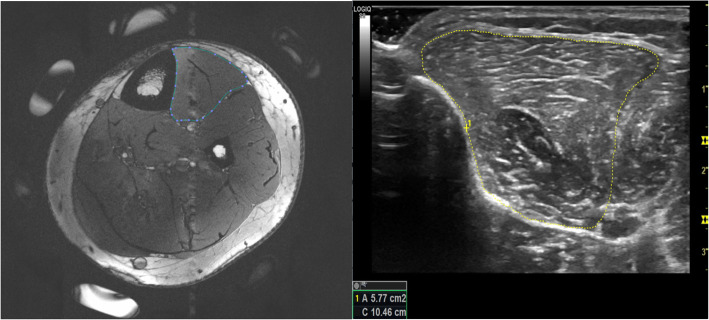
MRI (left) and US (right) images of the tibialis anterior muscle with associated segmentation of the muscle
